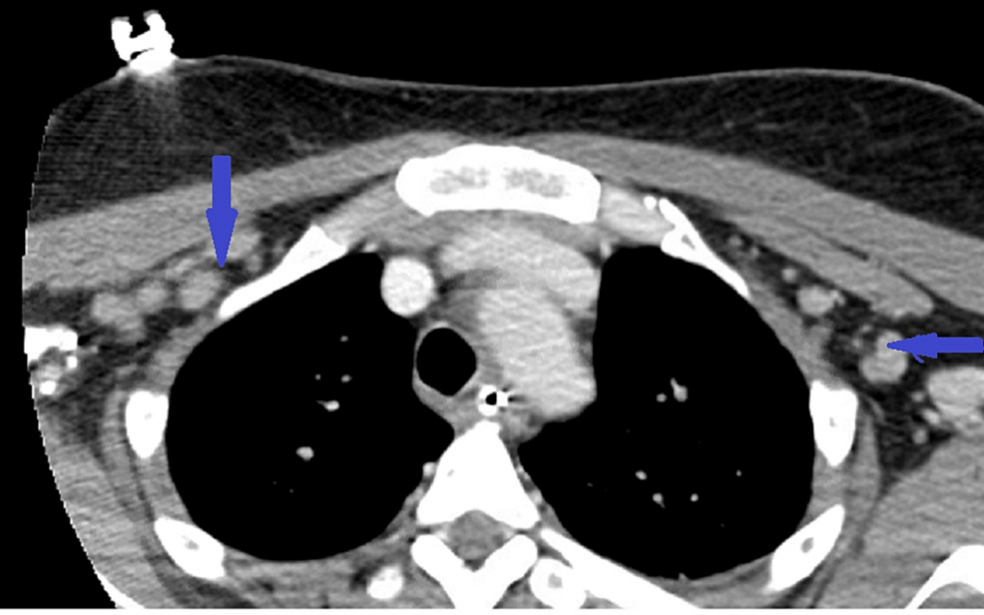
Bilateral axillary and pre-pectoral lymphadenopathy was noted on CT chest (blue).
